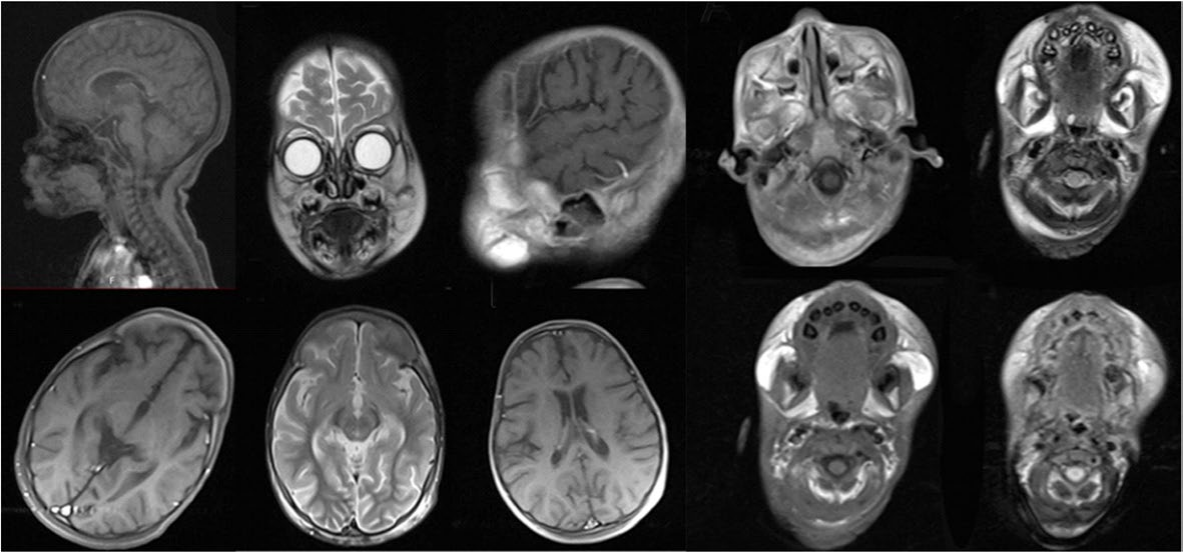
Head and neck MRI findings. No specific abnormal findings were seen in MRI. The condition of cerebral ventricles and other components is normal
